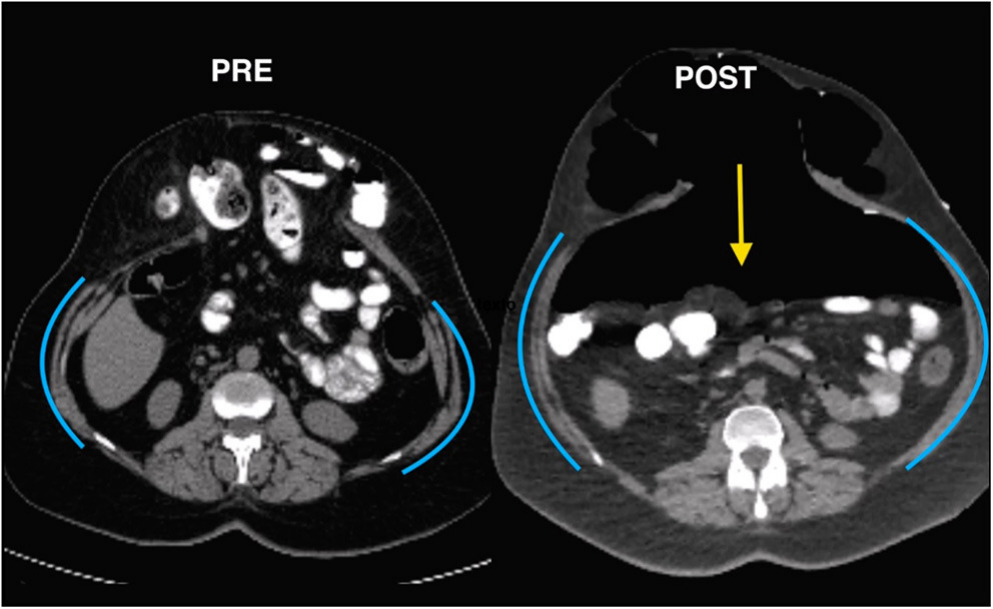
Changes in abdominal volumes and hernia diameters in CT after BTA and PPP.
